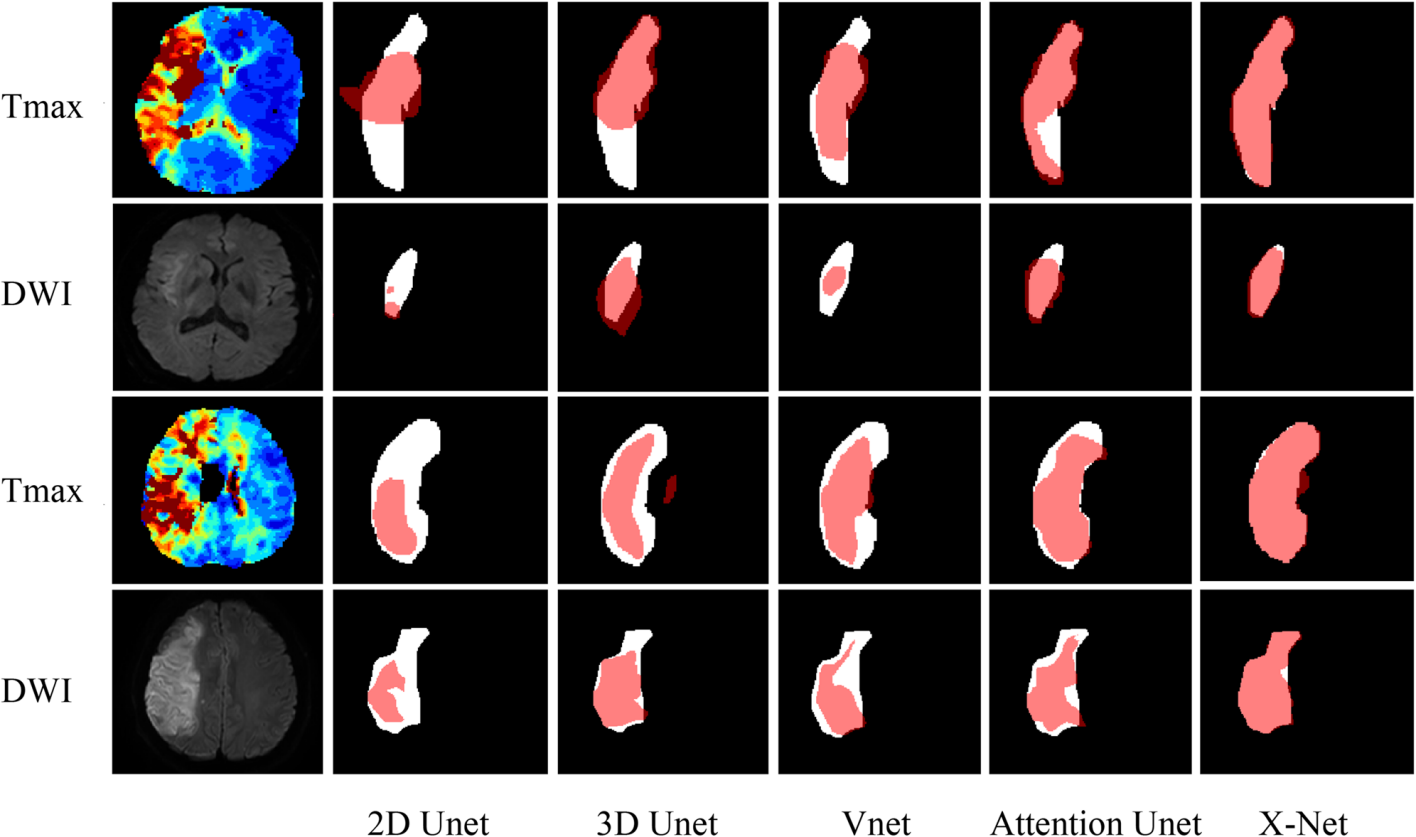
Visualization of the segmentation ischemic core in the DW images and the abnormal perfusion area in the Tmax images by using different networks, with the X-Net model showing the best performance. White regions represent the manually outlined VOIs, and red regions represent the automatically segmented VOIs. DWI = diffusion-weighted imaging, VOIs = volumes of interest
